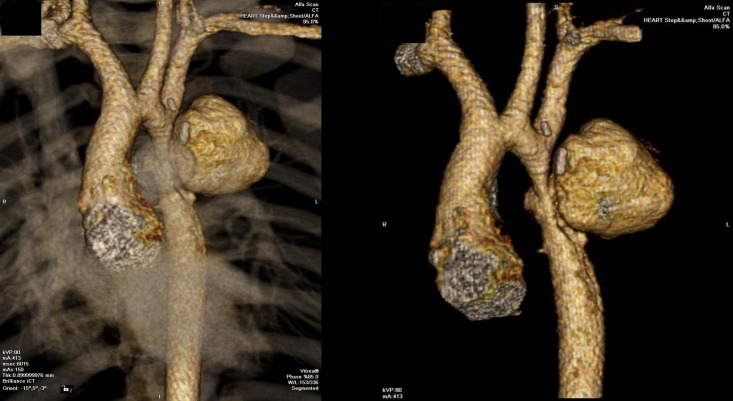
Multi-slice CT aortography showing an aortic pseudoaneurysm at the site of surgical coarctation repair in a 6-year old child.
